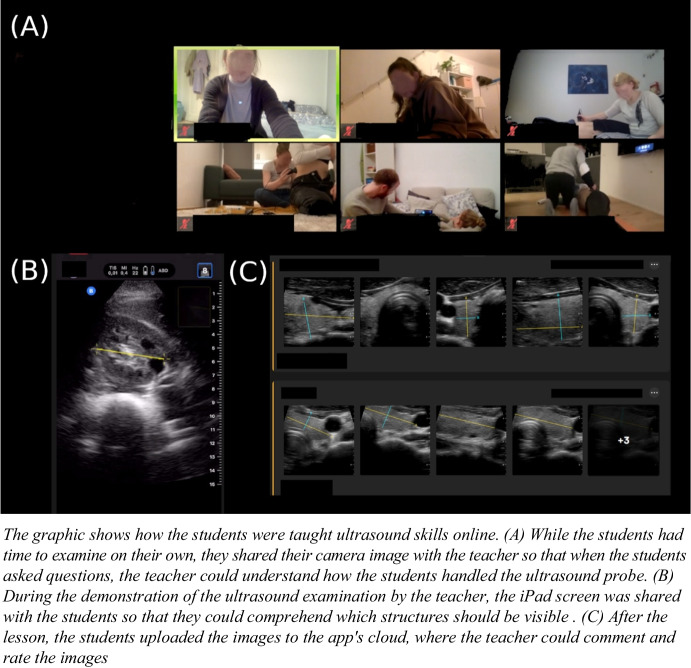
Demonstration of the implementation of the teledidactic ultrasound course.
